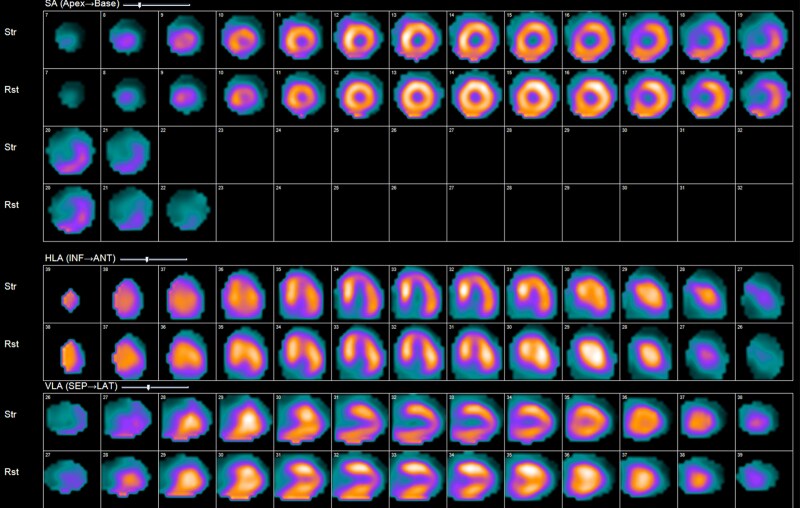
Slice-level images from the Case 2 single photon emission computed tomography.
